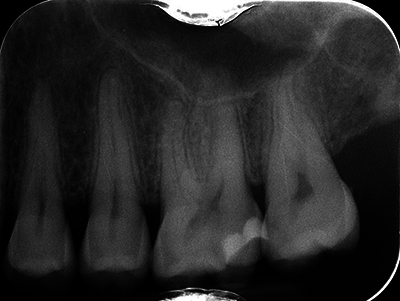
Radiography retro-alveolar post treatment (note the new densification around teeth).
